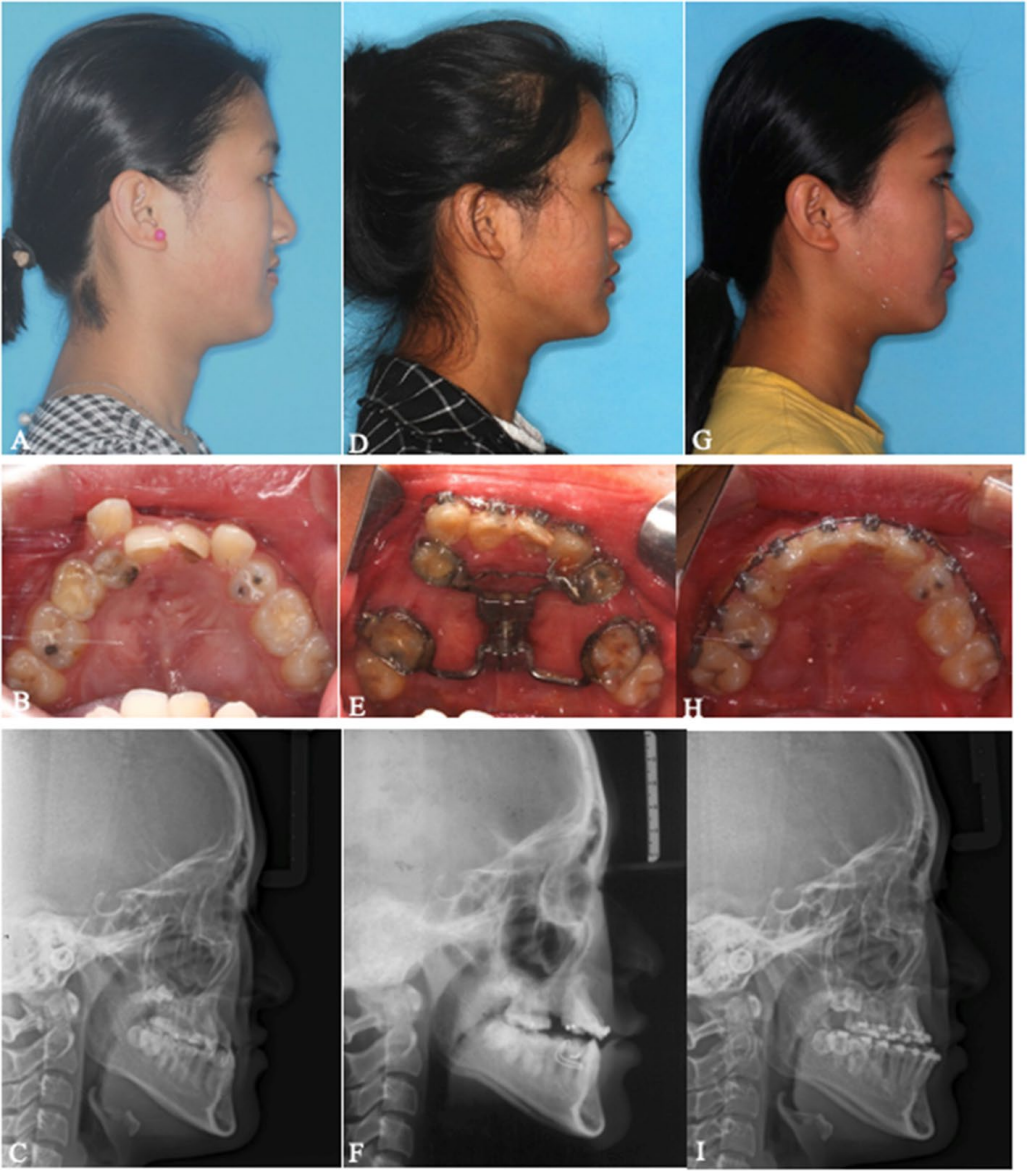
A-C Photographs, intraoral view and lateral cephalometric radiograph before treatment. D-F Photographs, intraoral view and lateral cephalometric radiograph after the consolidation period. G-I Photographs, intraoral view and lateral cephalometric radiograph after preoperative orthodontic treatment. MASDO was applied and the osteotomy line was drawn on the palate between the molars and premolars on both sides. One mini-screw was inserted in the planned position. Loosening of the mini-screw occurred at 12 weeks after the osteotomy. The premaxilla moved forward and the facial profile was improved after MASDO. We initially planned to alleviate dental crowding and create space for the restoration of No.15 and No.25 using the space produced by the MASDO produce. However, the patient wished to close the space without restoration, so the distracted space was subsequently closed by mesializing the molars
